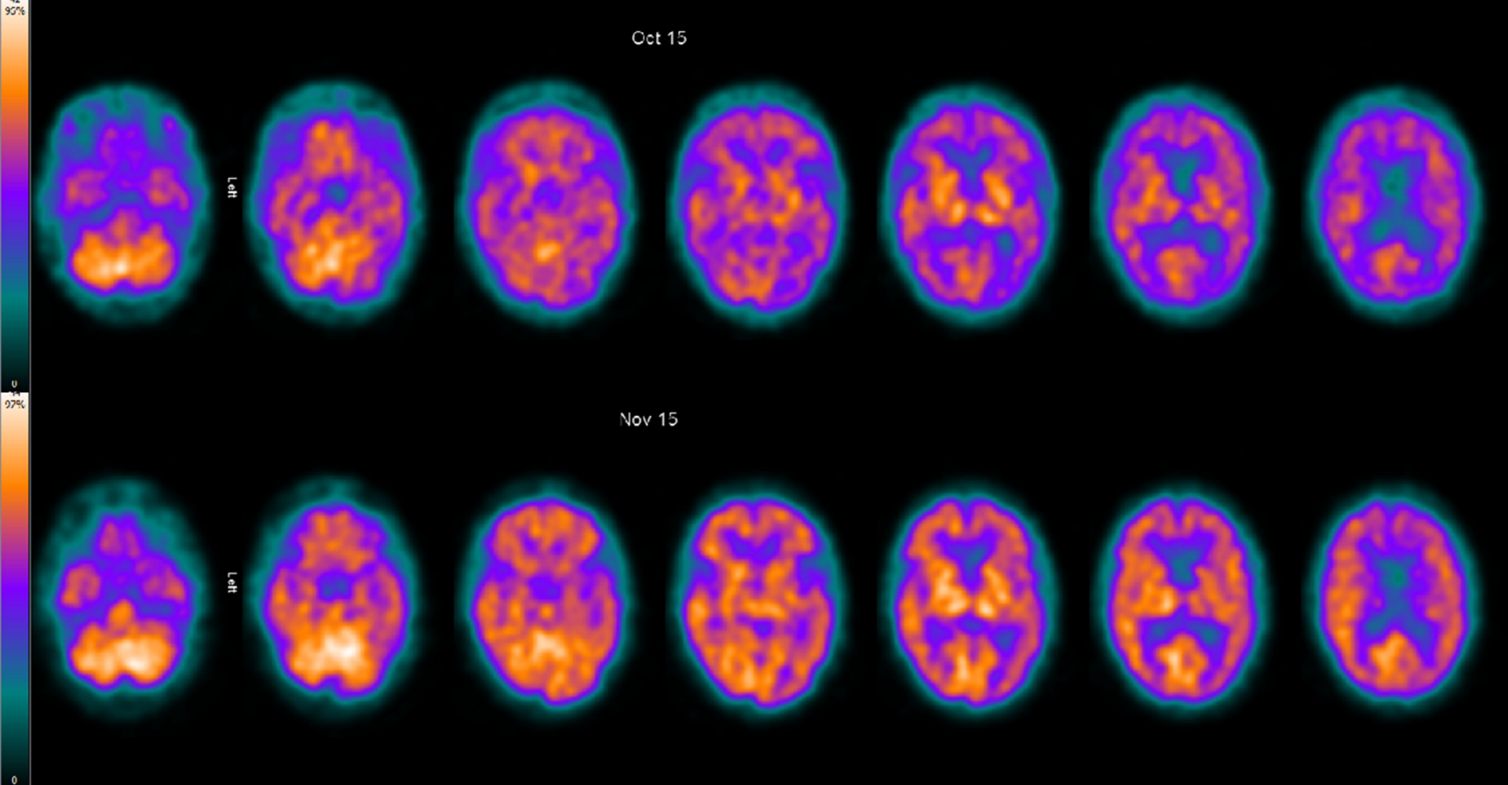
Cerebral perfusion single photon emission CT using intravenously injected ^Tc^99m HMPAO. Axial projections. Pretreatment (top row) and post-treatment (bottom row) scans. As shown in the colour scale to the left, the brighter yellow the colour the greater the perfusion. Cortical perfusion is generally reduced on the pretreatment scan, but significantly improved after treatment.
